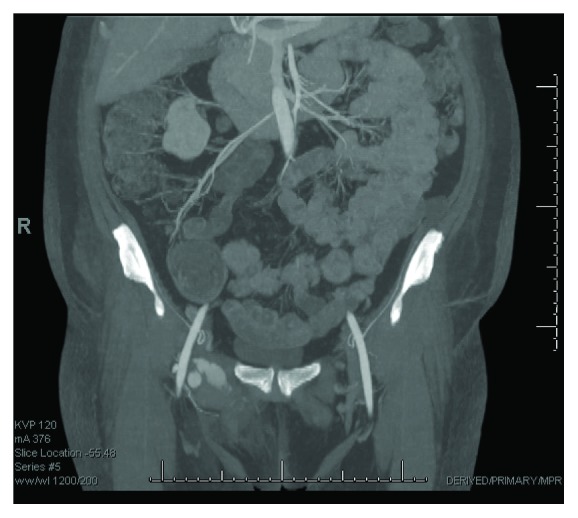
CTA showing right common femoral artery pseudoaneurysm.
